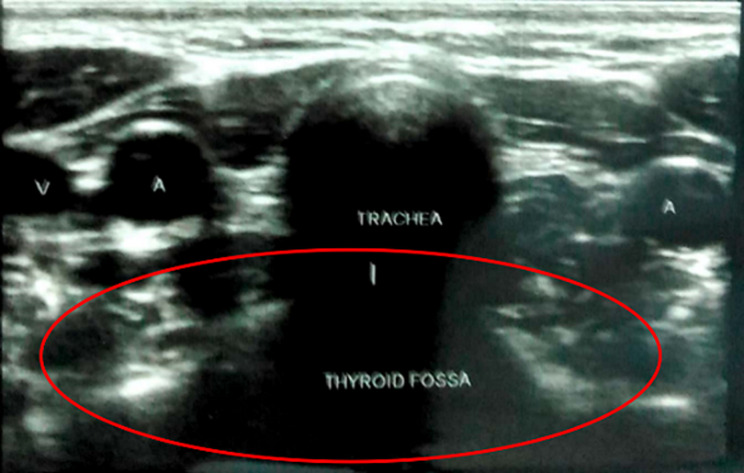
ultrasound of the neck revealed no thyroid formation in the thyroid fossa (red circle)
